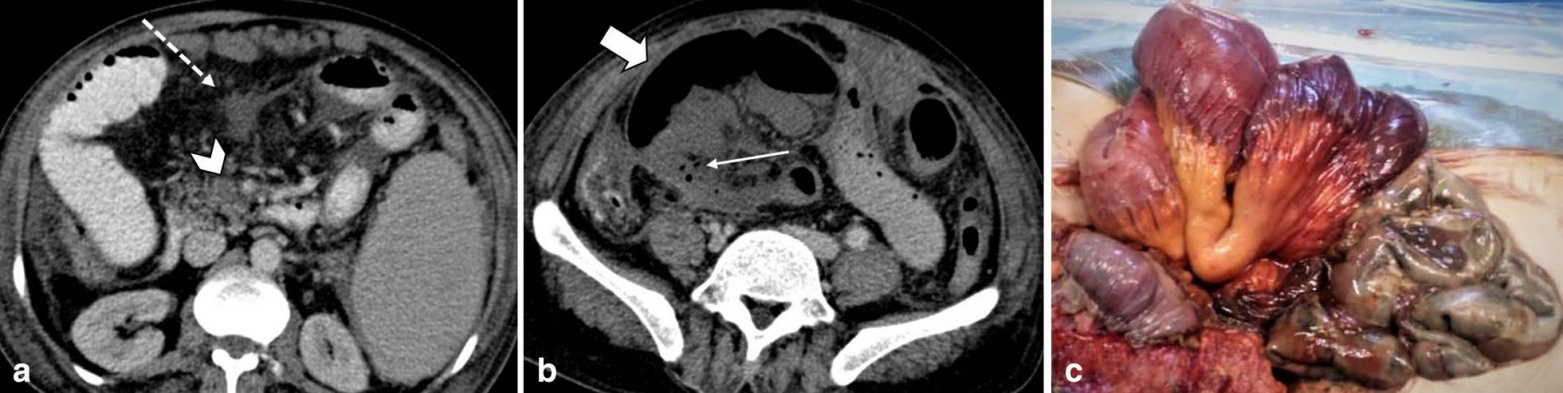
A 66 y/o cirrhotic male patient presenting with acute SMV thrombosis. a, b CT images reveal complete filling defect in SMV (arrow head) and mesenteric fluid (dotted arrow) associated with mural pneumatosis (thin arrow) and thinning (thick arrow) in a dilated ileal segment. c Intra operative image shows necrosis of ileal segments along with some adjacent borderline segments
